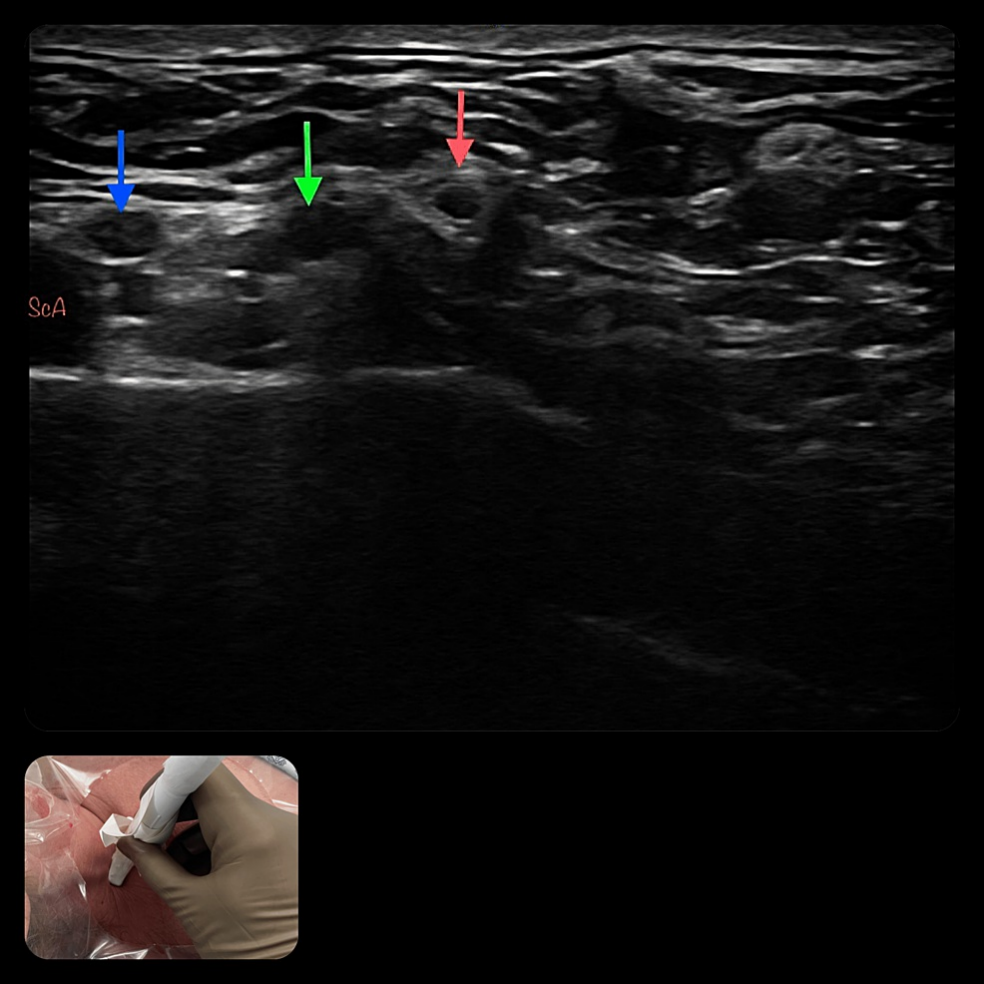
Ultrasound probe position and image for anterior suprascapular nerve block ScA: subclavian artery, Blue arrow: anterior division of upper trunk, Green arrow: posterior division of upper trunk, Red arrow: suprascapular nerve
